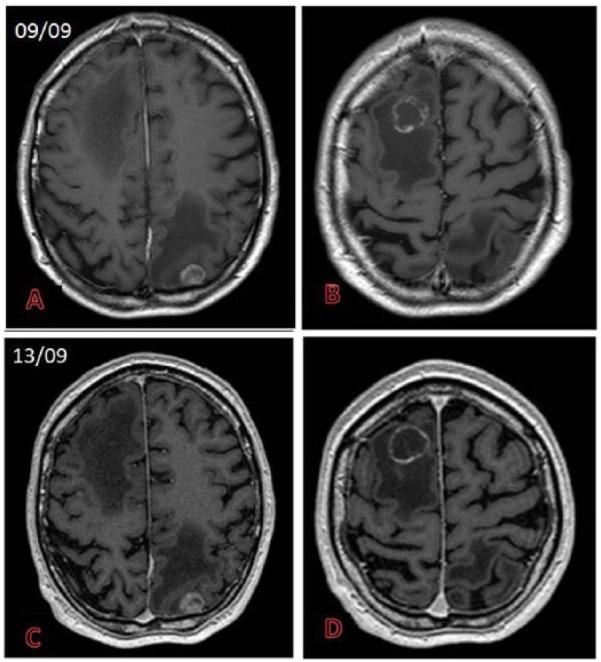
Multiple brain metastasis from lung cancer with ring-like enhancement and surrounding edema (A-D: postcontrast T1-weighted sequences) in the right frontal and left occipital lobes studied within an interval of 4 days (September 9 and September 13) with different contrast agents ( 13 mL of gadoterate meglumine in A and B and 6.5 mL of gadobutrol in C and D), with the same postcontrast delay. Better and more consistent enhancement seen in C and D due to the higher relaxivity of the contrast agent.
